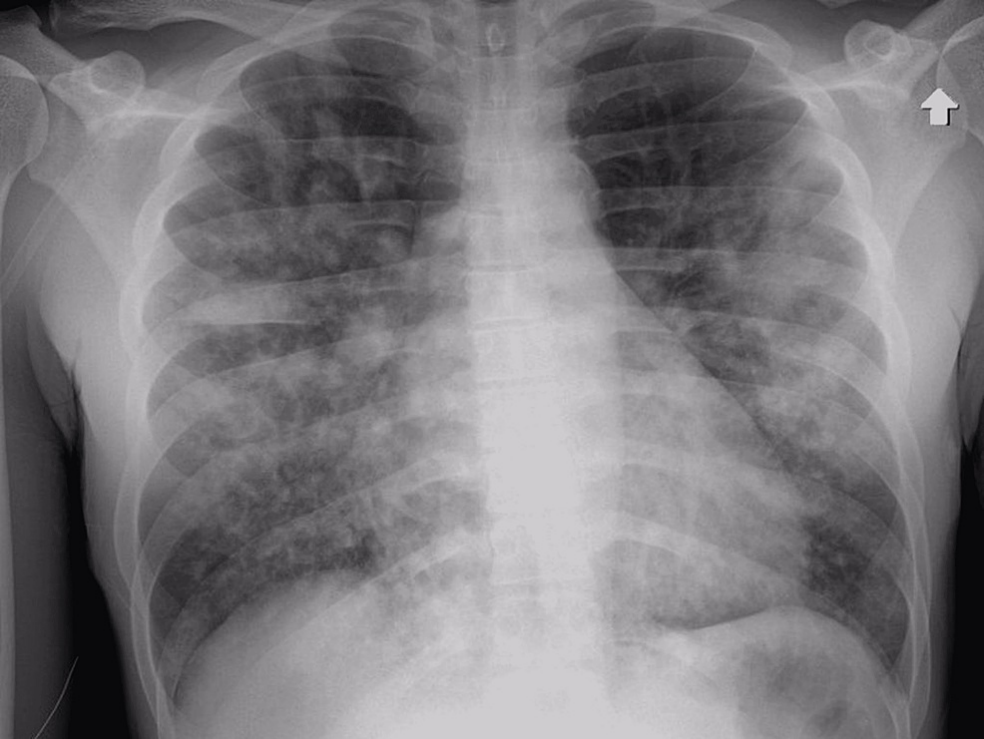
Chest radiography showing diffuse multifocal nodular opacities
